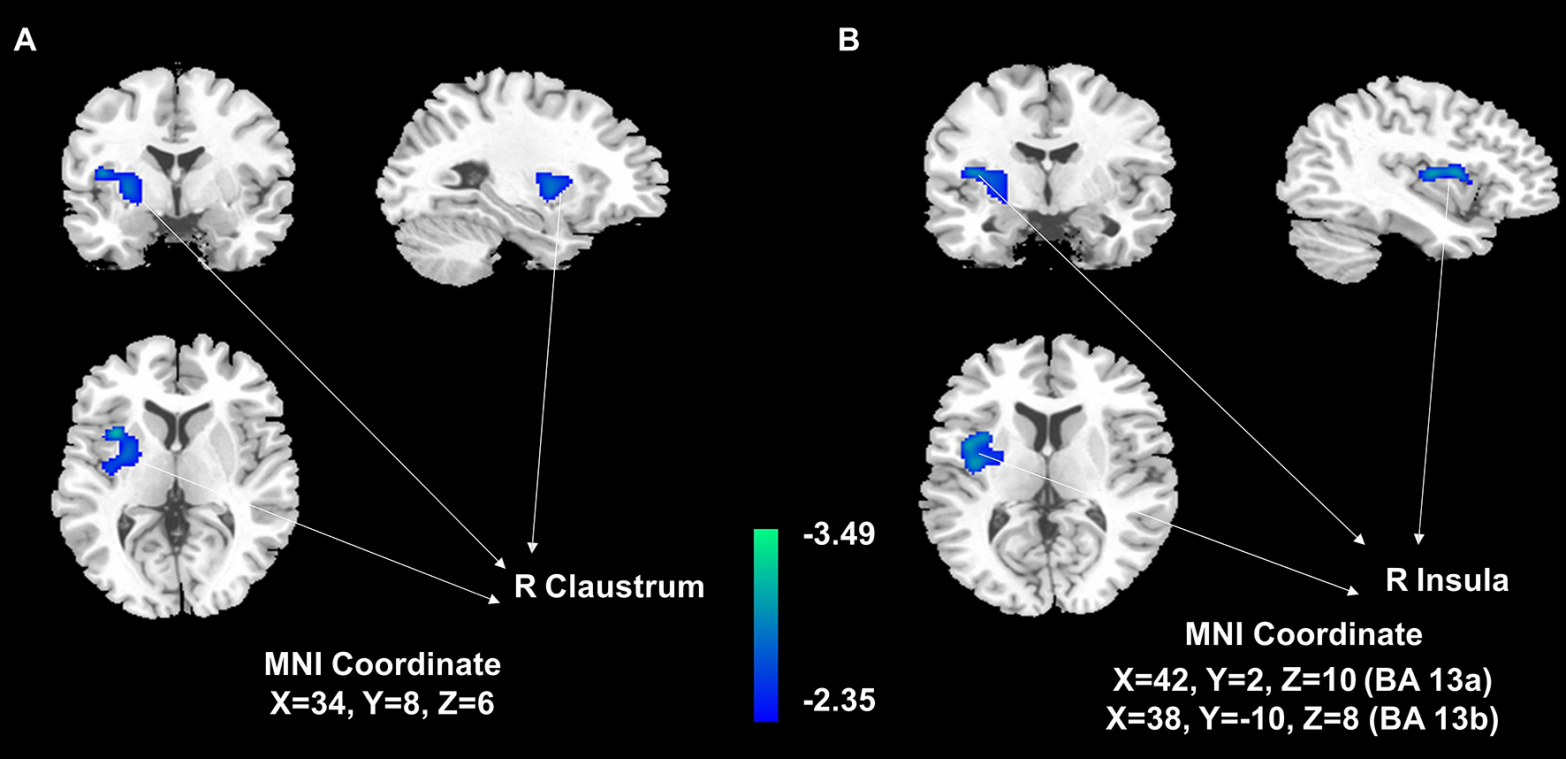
Areas of significant differences of glucose metabolism in subjects with activated BAT, compared with controls. Statistical Parametric Mapping (SPM) results: It is displayed on a T1 template overlaid with magnetic resonance images at the threshold of uncorrected *P*<0. 01, threshold K>321.6, and the gray-scale figures are a T1 structural MRI that are representative of MNI space. The blue areas represent relatively hypometabolic regions of decreased ^18^F-FDG uptake in the right claustrum (A) and right Insula (B) in BAT-positive subjects than in negative control subjects.
